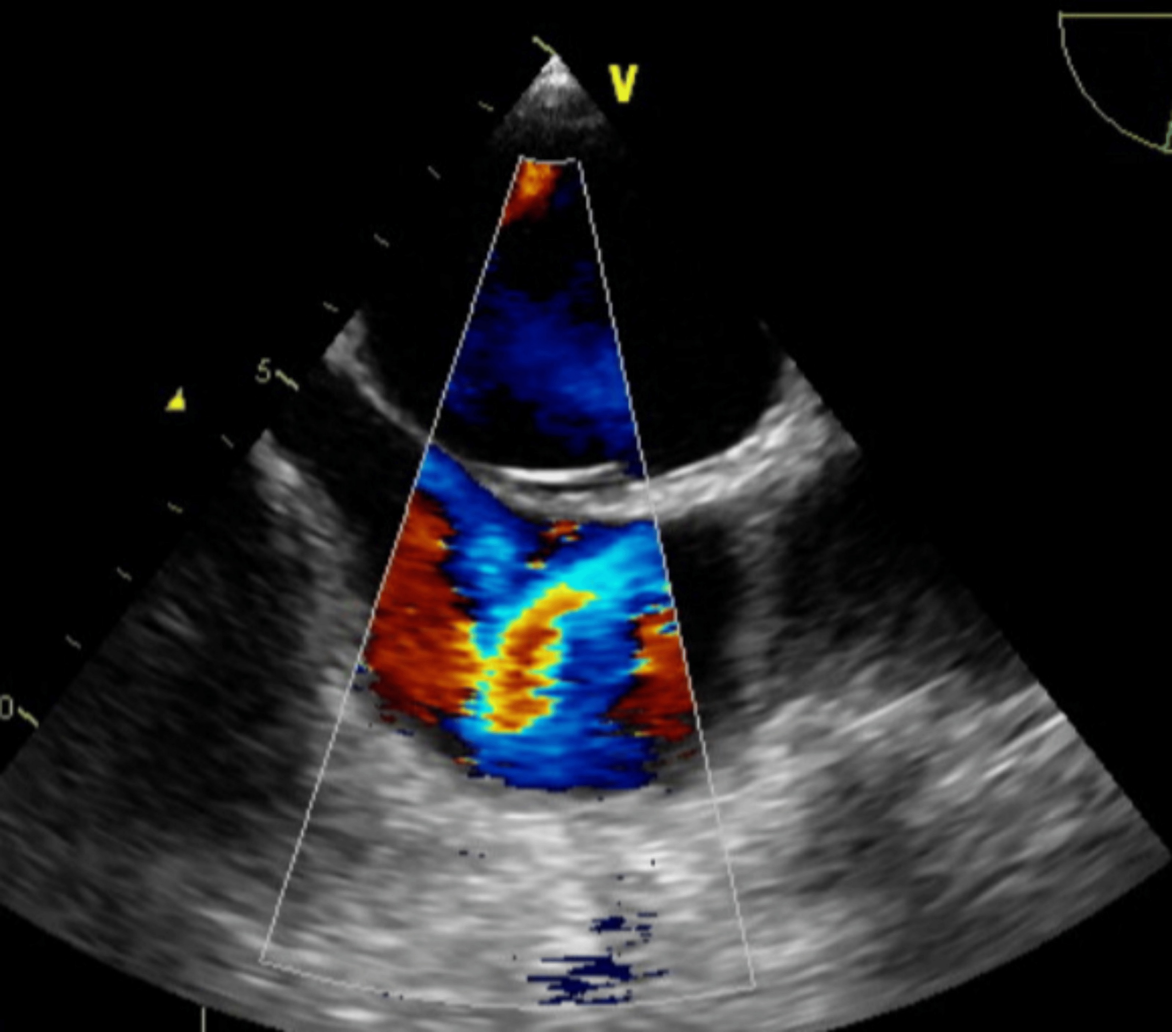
Color doppler on TEE showing severe mitral regurgitation. TEE: transesophageal echocardiogram
